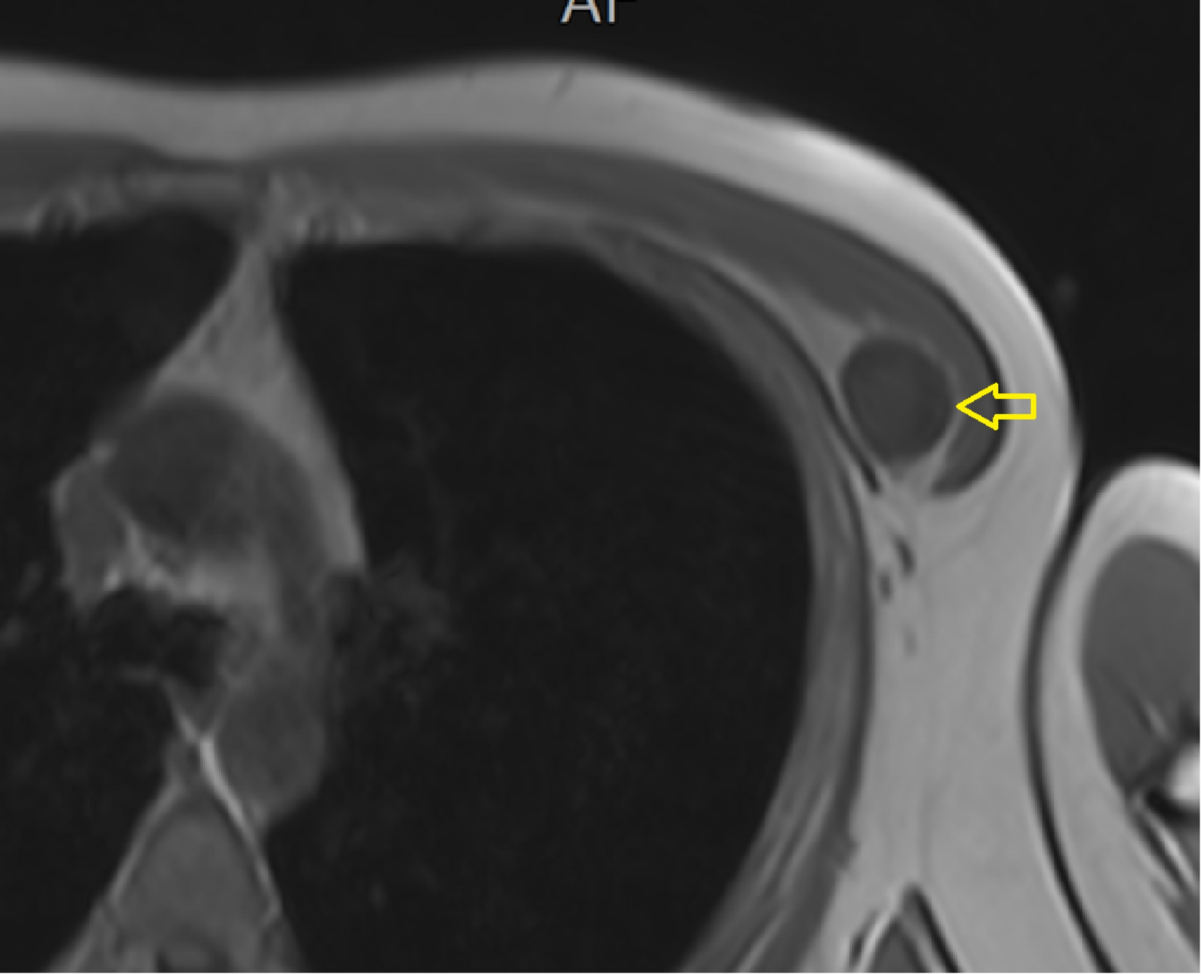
T1-weighted MRI of the chest Isointense, well-defined lesion (arrow) within the intermuscular plane intervening between the left-sided pectoralis major and pectoralis minor muscles. MRI: magnetic resonance imaging
